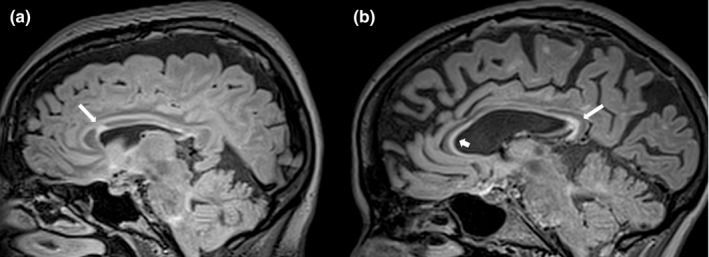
Parasagittal FLAIR images showing the appearance of Corpus Callosum lesions in a patient with Fabry Disease (a) and in one with Multiple Sclerosis (b). In the MS patient is possible to better appreciate, compared to FD, the typical appearance of the calloso‐septal lesions, which are defined as narrow hyperintense bands along the undersurface of the corpus callosum itself
